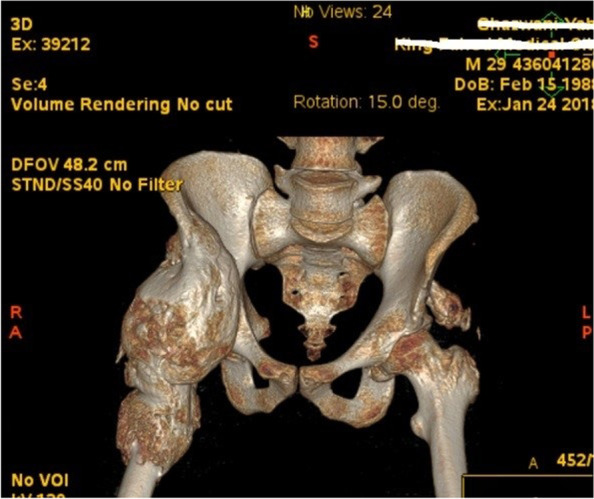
Patient with heterotopic ossification following brain injury. (3D osseous CT showing G2 Lt hip heterotopic ossification and G4 RT hip heterotopic ossification for a patient with long-standing bed bound stay following brain injury)
